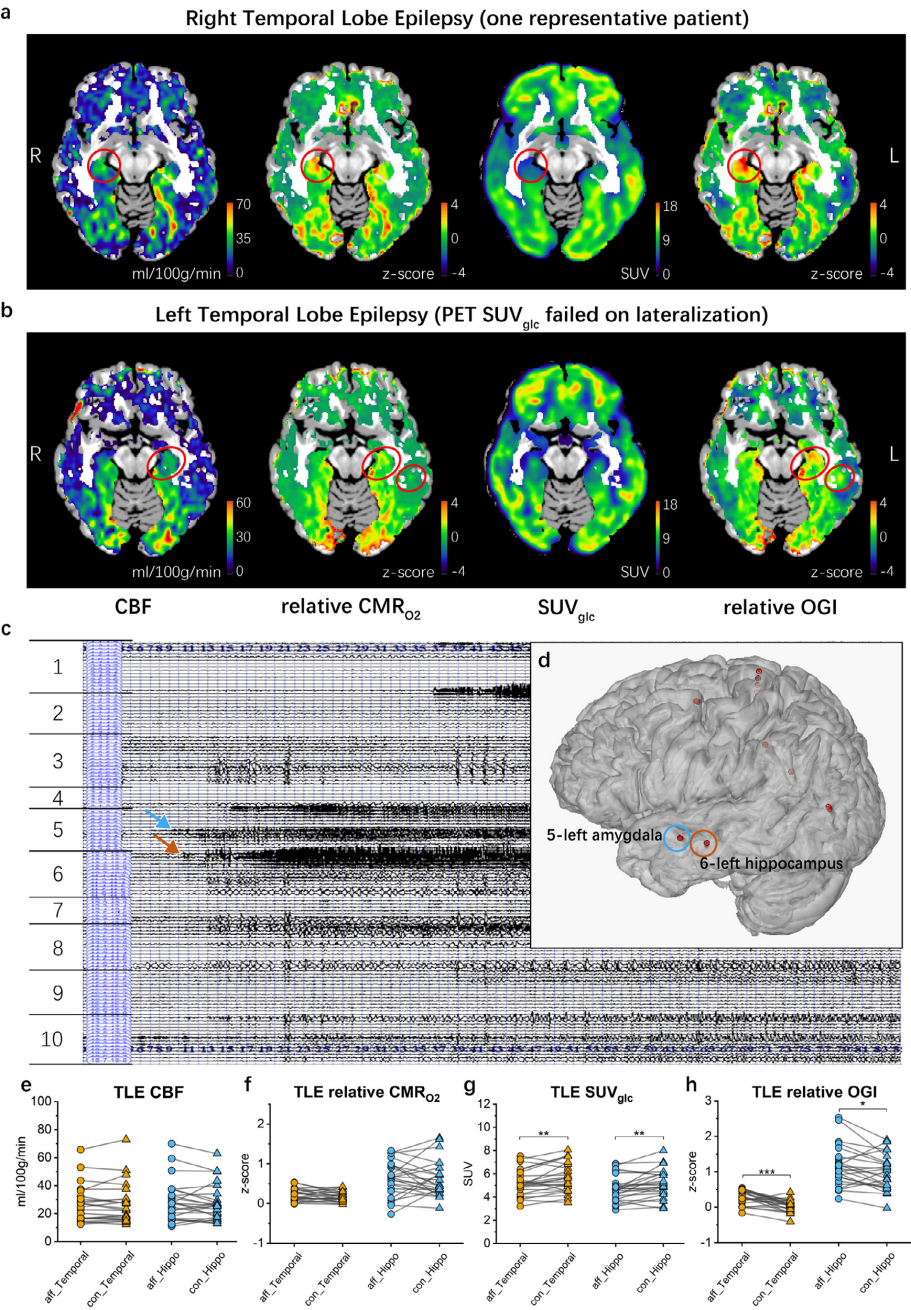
Representative patients with temporal lobe epilepsy and statistics across different brain regions. (a) Maps of CBF, relative CMR_O2_*z*-scores SUV_glc_ and relative OGI *z*-scores (as labelled in b) from one typical temporal lobe epilepsy patient where the SEEG, PET and OGI all identified the epileptic focus on the right side. The red circle indicates the abnormal values in the hippocampus on the affected right side of the brain. (b) Maps of CBF, relative CMR_O2_*z*-scores, SUV_glc_ and relative OGI *z*-scores from one temporal lobe epilepsy patient, in which PET results were inconclusive, but in which SEEG identified the epileptic focus on the left side. The red circle indicates the abnormal values from this study on the affected left side, which agree with the SEEG results. (c) SEEG trace of the seizure origin for the patient from b. Ictal SEEG recording showed that continuous, repetitive spikes arose from the left hippocampus (brown arrow) and left amygdala (blue arrow) and spread to the central area. The indices indicate the brain region as described in Supplementary Table 2. (d) Left lateral view of the reconstructed 3D MRI brain image of the patient from b. The positions of the right hemisphere electrodes are shown in red, co-registered with the MRI image. The red dots circled in blue (left amygdala) and brown (left hippocampus) show the position of the electrodes that detected abnormal discharges. (e-h) Mean CBF (e), relative CMR_O2_*z*-score values (f), SUV_glc_ (g)*,* and relative OGI (h) *z*-score values of the left and right temporal lobe and hippocampus. The differences of the population mean on the left and right brain regions in SUV_glc_ and relative OGI are significantly different using pair-sample *t*-tests, 0.001<*p*<0.01, p*<0.001. In SUV_glc_ (g), *p* = 0.0098595 for temporal lobe, *p* = 0.0091322 for hippocampus. In relative OGI (f), *p* = 1.5594×10^−5^ for temporal lobe, *p* = 0.029193 for hippocampus.
